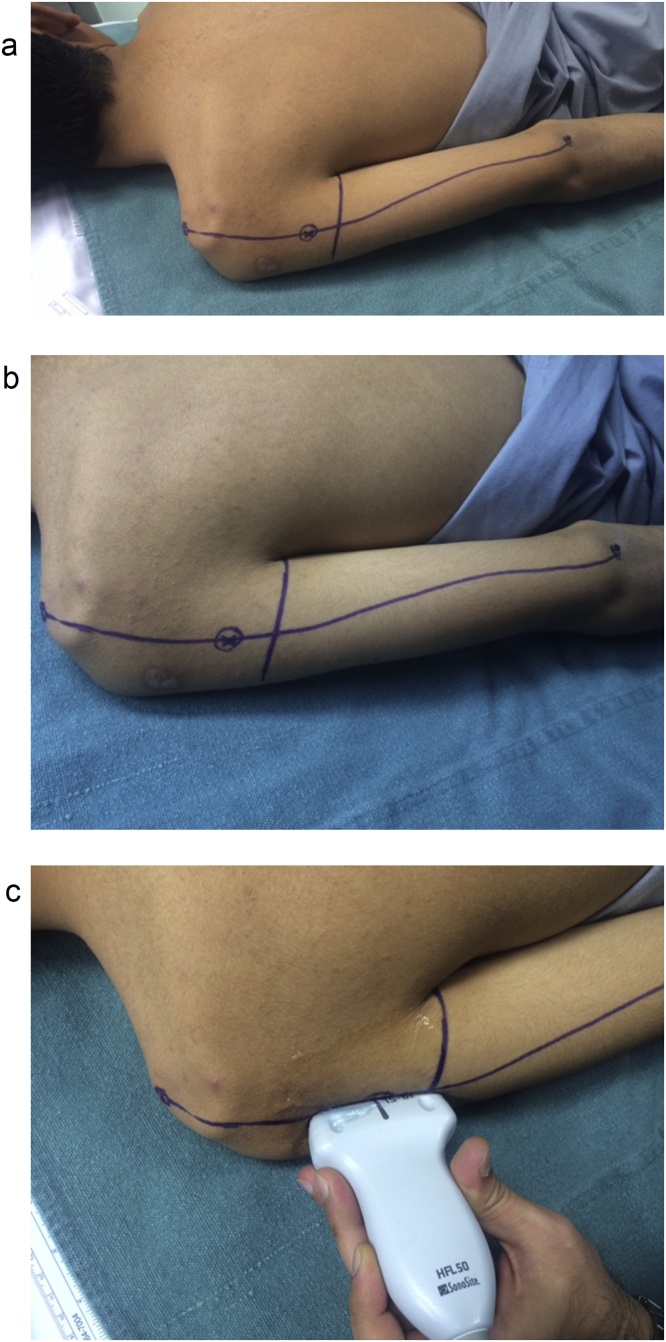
a) The longitudinal acromion – olecranon line transecting the transverse line from the axillary fold. Anatomically, the quadrilateral space is 2 cm cranial to this transection point. b) A close up of the two lines and the point (x) localizing the quadrilateral space. c) The ultrasound probe is use.
